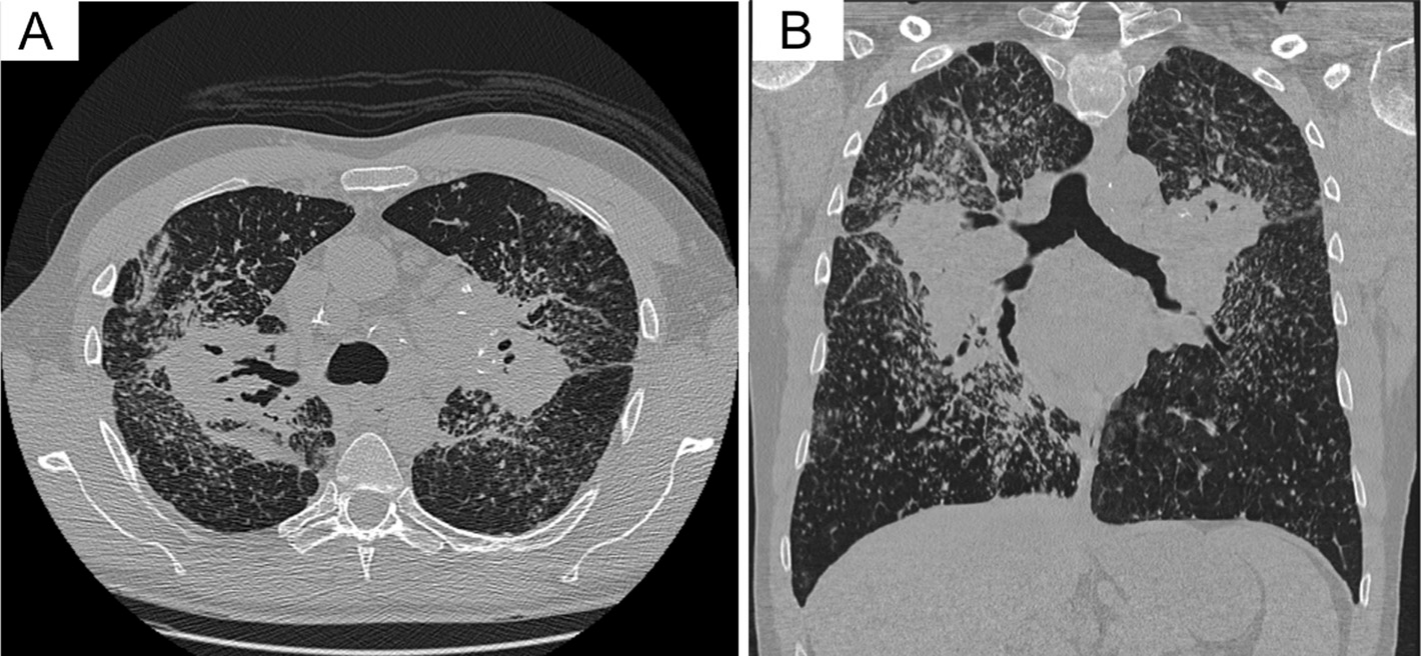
Selected axial (A) and high resolution coronal (B) reformats from a non-contrast helical CT shows striking bilateral bulky hilar and mediastinal adenopathy. Mass-like fibrosis is encasing the hila and central bronchi causing traction bronchiectasis. There are several partially calcified hilar lymph nodes. There are also extensive perivascular and perilymphatic nodules throughout both lobes with relative sparing of the lower zones and right upper lobe bullae.
